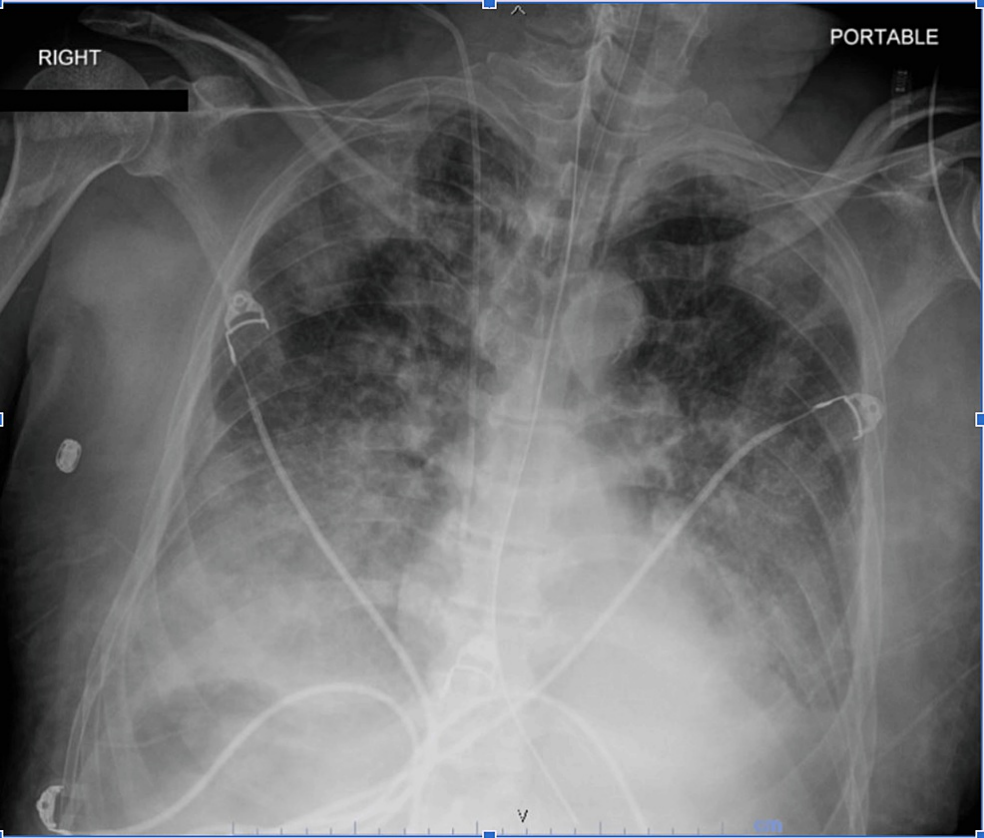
Chest X-ray demonstrating ARDS in our patient. ARDS: acute respiratory distress syndrome.
